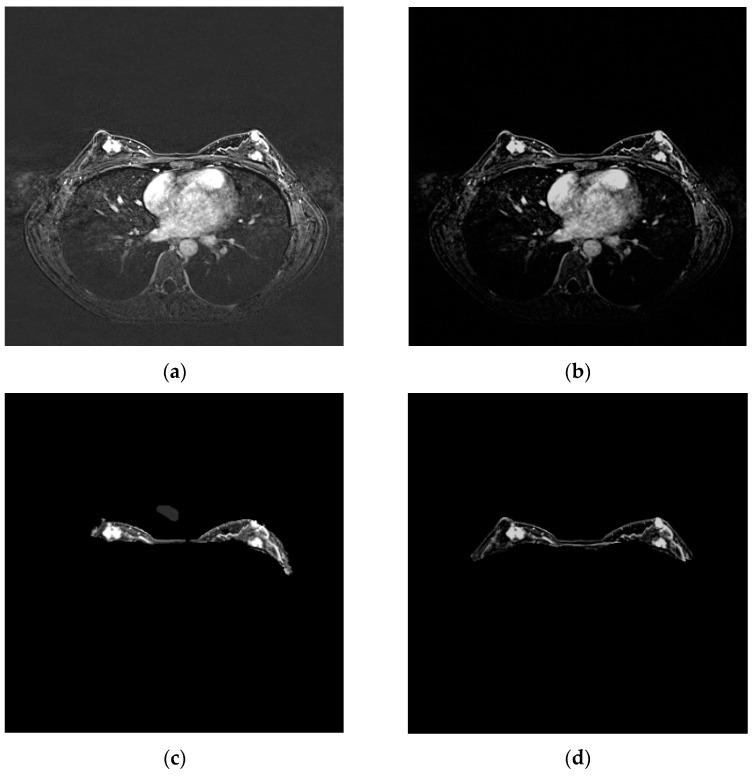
Background noise reduction: (a) MRI before noise reduction; (b) MRI after noise reduction; (c) segmented breast of original MRI; (d) segmented breast of noise reduction MRI.
